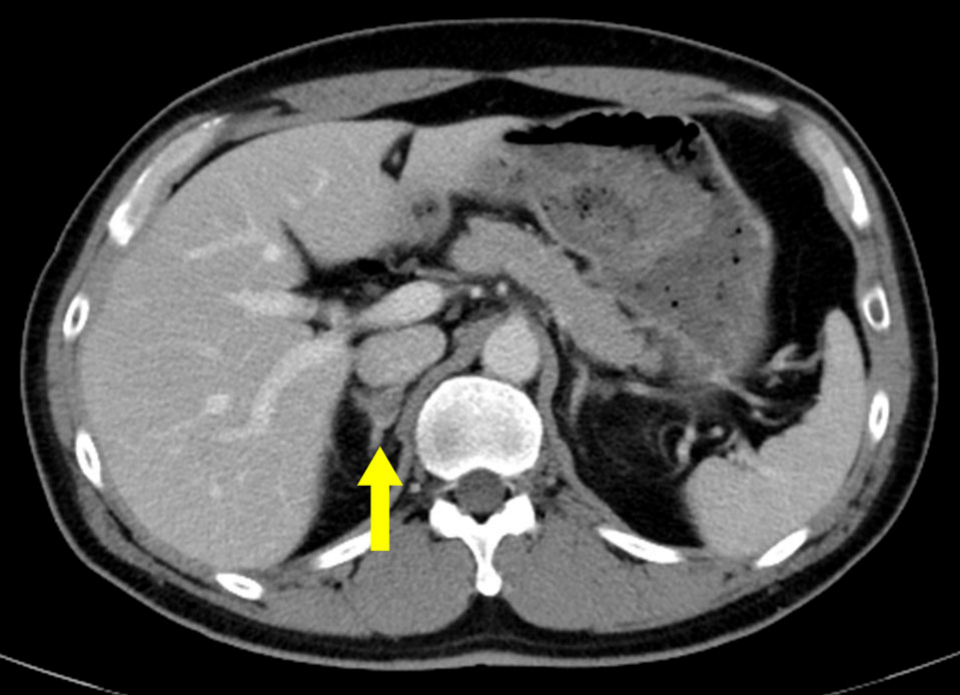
Enhanced computed tomography before adrenalectomy. Right adrenal metastasis remains after local radiotherapy and multiple chemotherapies.
